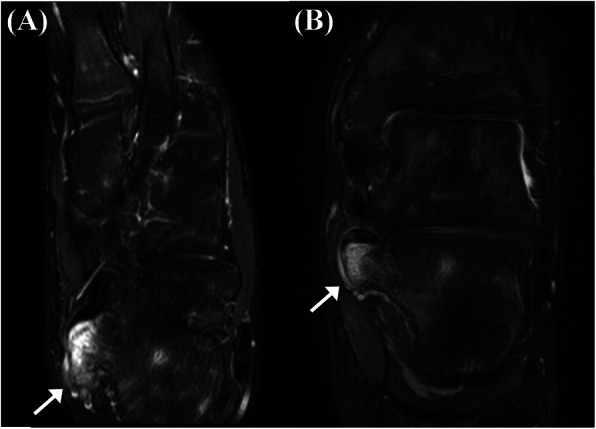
Preoperative MRI scans show the high T2 signal intensity mass extended from the sustentaculum tali occupying the tarsal tunnel (a, b). The fatty bone marrow is seen on the central portion of the mass, continuously with the marrow cavity of the underlying bone, surrounded by thin cartilaginous cap (arrows)
